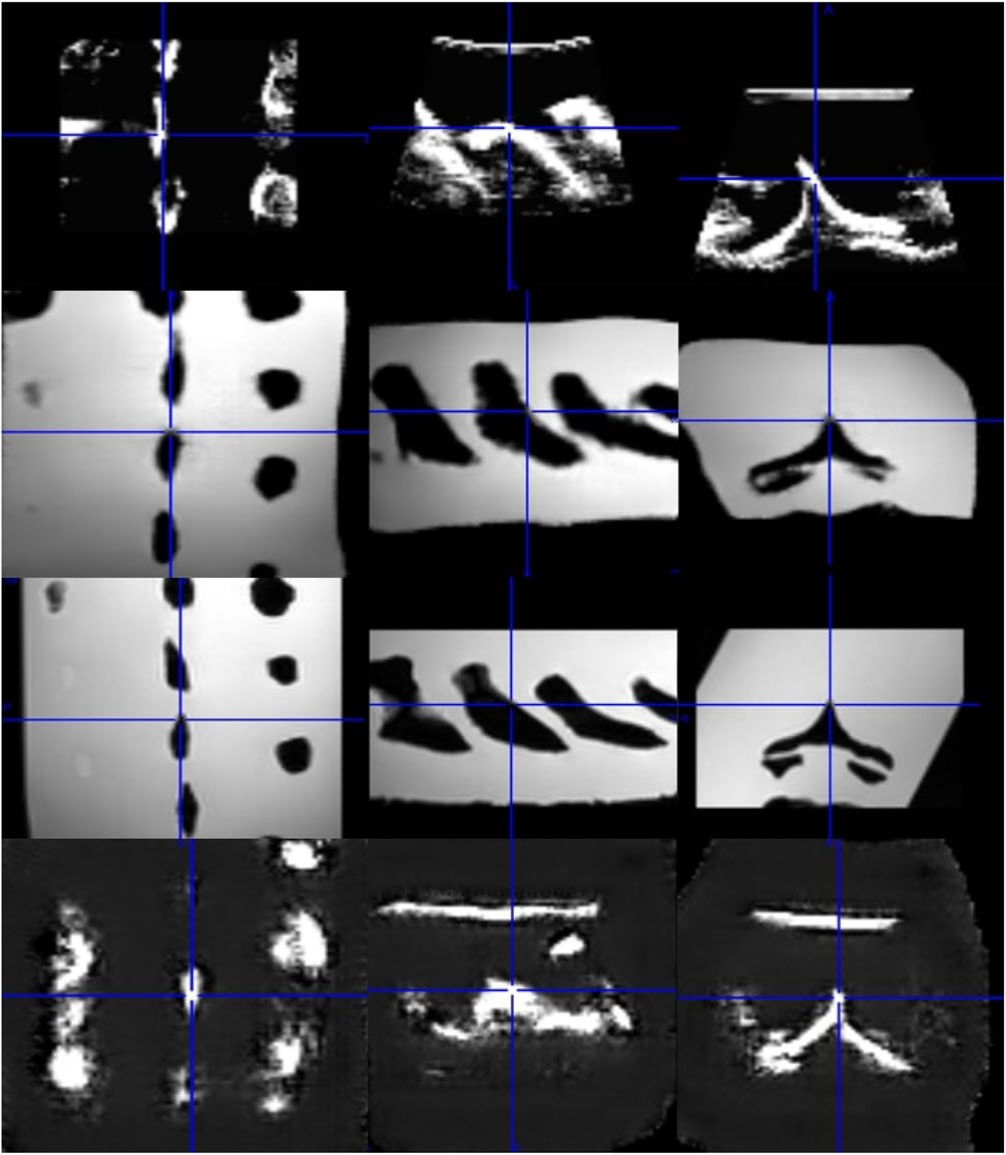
The rows (from top to bottom) represent the real US, generated MRI, real MRI, and reconstructed US respectively. The columns (from left to right) show the posterior coronal, sagittal, and transverse planes respectively.
